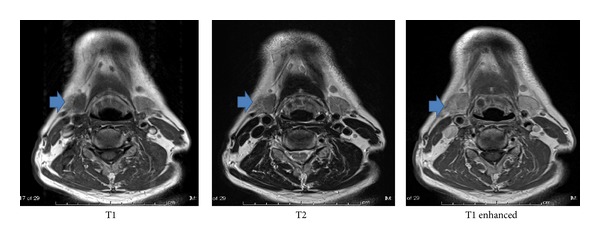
Neck MRI showing 20 mm × 10 mm tumor with an indistinct boundary and slight enhancement in the right submandibular gland. Signal intensities are low on both T1- and T2-weighted images.
